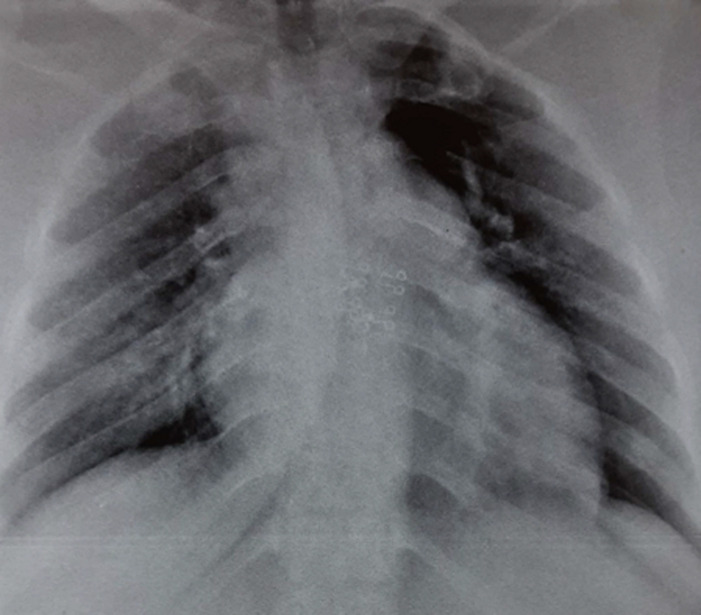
chest X-ray showing right basal pulmonary opacity, cardiomegaly at the expense of the right cavities, convex left middle arch
